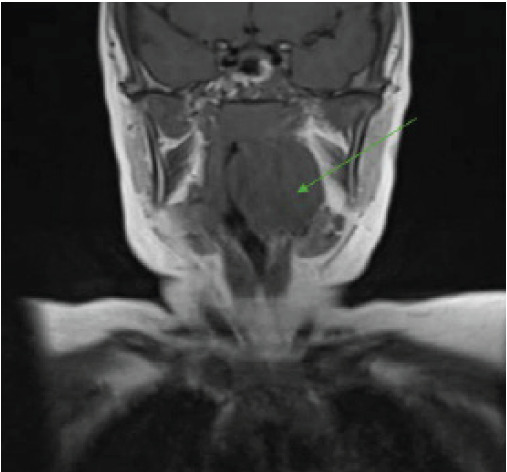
Magnetic resonance imaging coronal view depicting oropharyngeal mass (arrow).
